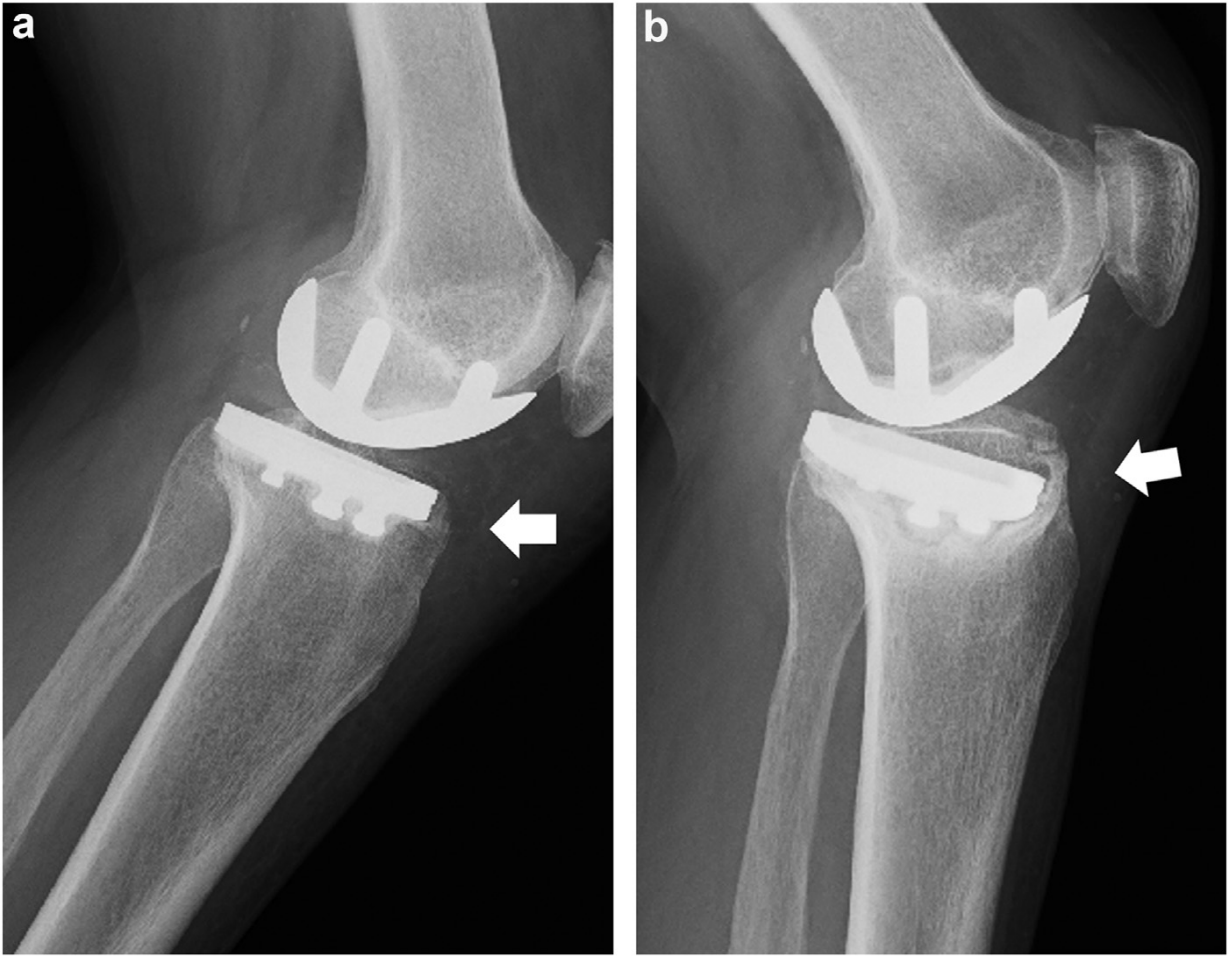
Case 2, 67-year-old female. (a) Postoperative radiography. Anterior tibial cortical support was not achieved (arrow). (b) Eleven months after surgery, collapse of the tibial component progressed (arrow).
